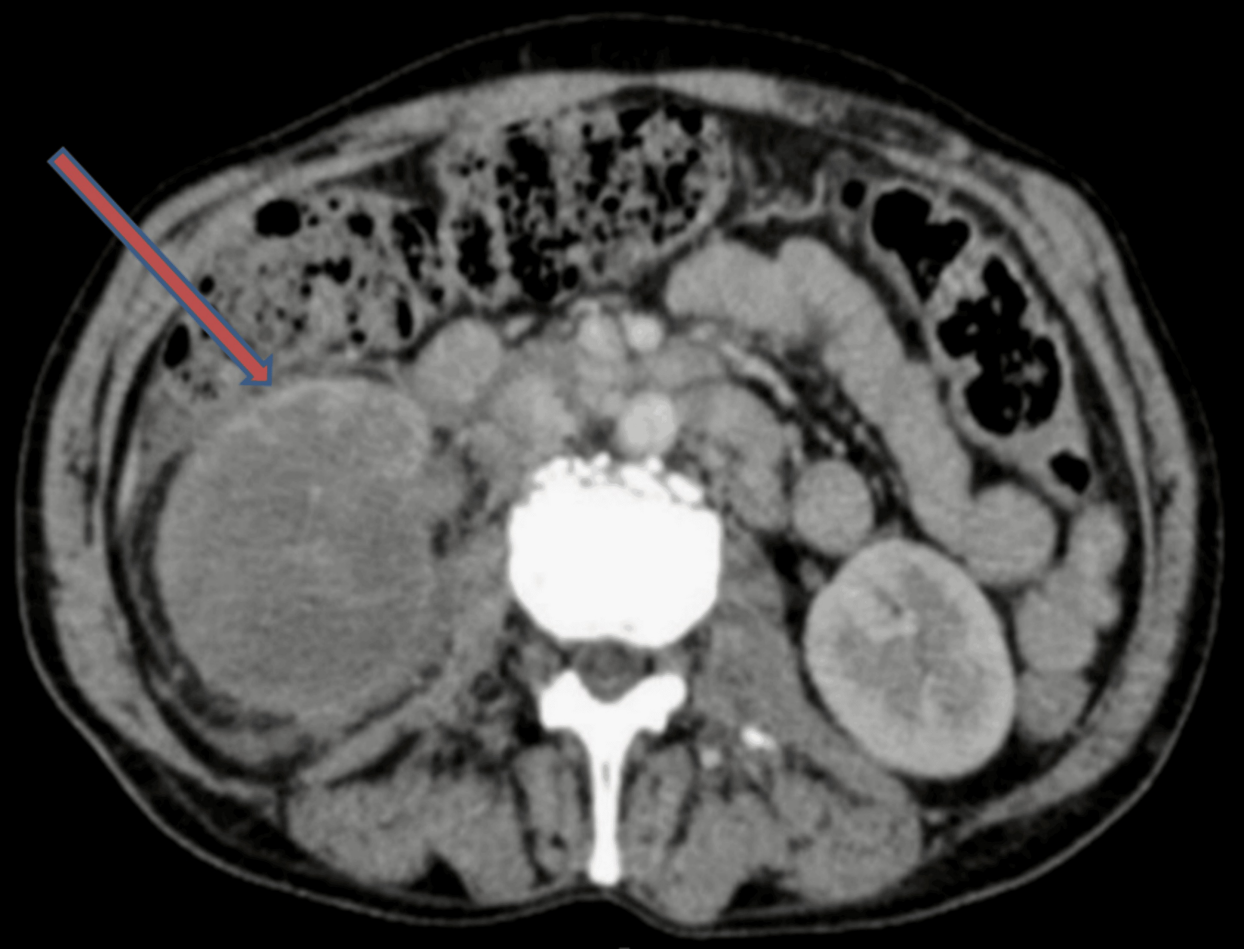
The axial contrast-enhanced CT image shows a peripherally enhancing mass with predominant hypoenhancement in the hilar and perihilar regions of the right kidney. This pattern of peripheral enhancement with central hypoenhancement is indicative of a malignant lesion, as it suggests the presence of a viable tumor rim with central necrosis or poor perfusion, characteristic of collecting duct carcinoma.
